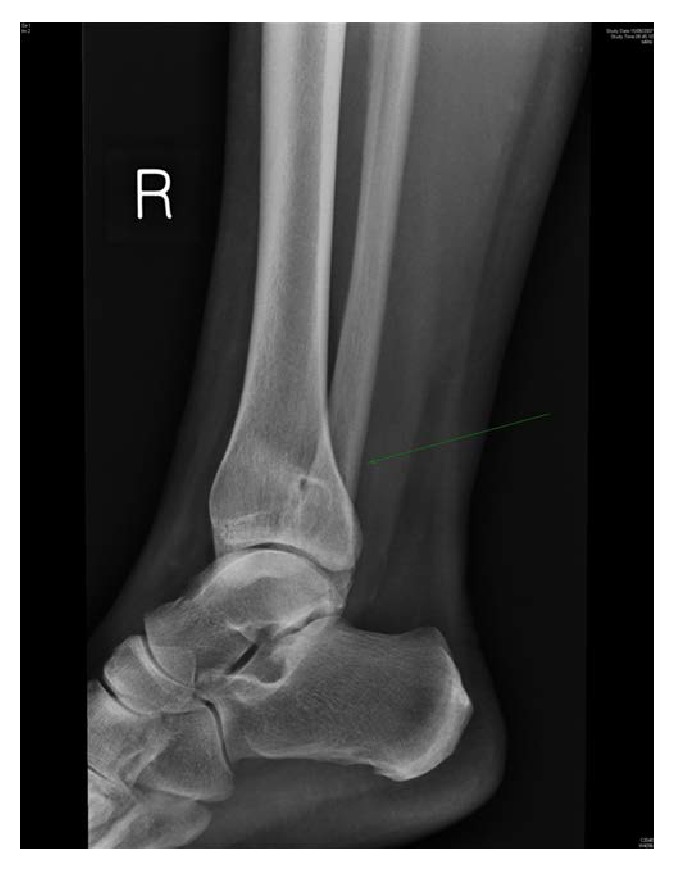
Lateral view X-ray of the ankle showing well defined cystic lesion in distal tibia.
